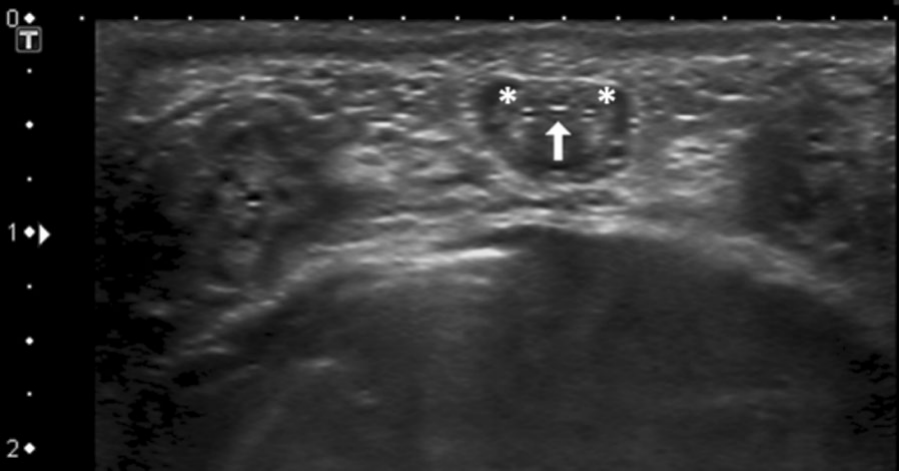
Transverse sonography of the penis of a 4-week-old ram. The urethra is flanked by two blood vessels allowing the examiner to orientate and find the urethra. *Arrow* transverse section of the urethra; *asterisk* flanking blood vessels
